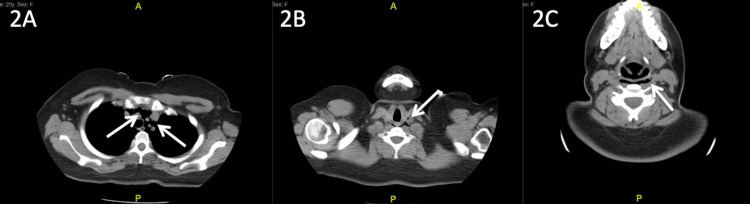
Non-contrast CT of the chest and neck (soft tissue window) on hospital day one Arrows point at subcutaneous air that can be seen in the superior mediastinum (2A), along the carotid sheath (2B), and retropharyngeal space (2C) CT: computed tomography
